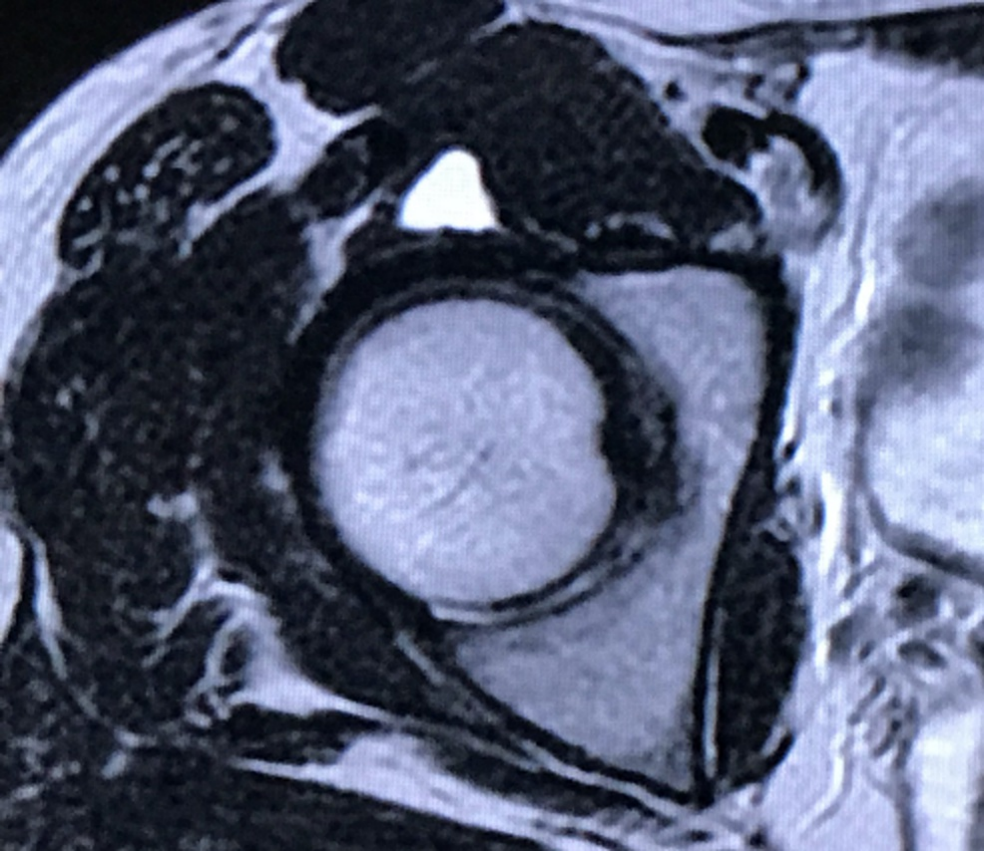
Preoperative MRI, axial view, shows the high-intensity mass between the iliopsoas muscle and anterior capsule.
